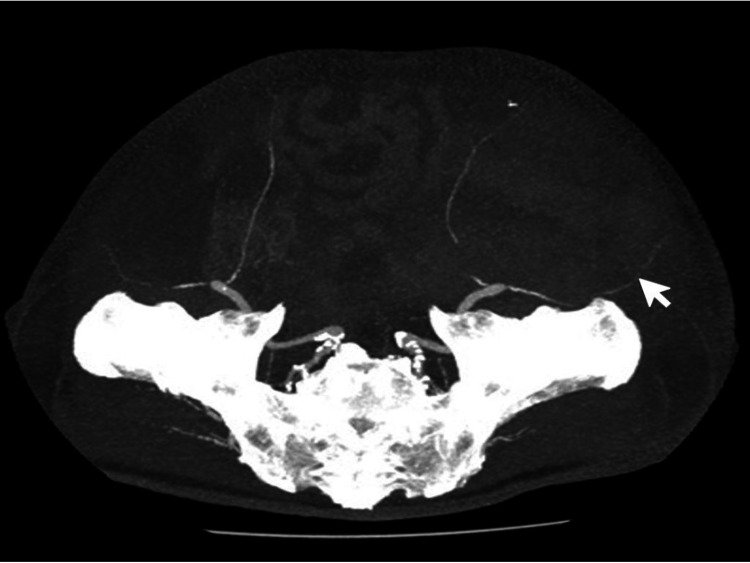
Computed tomography-angiographic (maximum-intensity projection) image of the arterial phase showing the course of the left deep circumflex iliac artery (white arrow) arising from the left external iliac artery.
